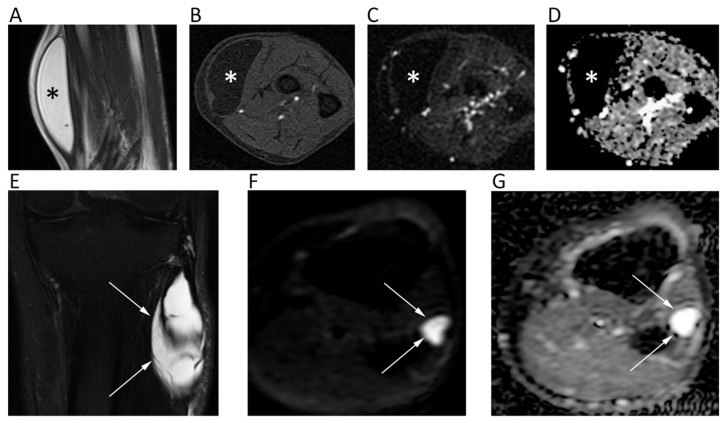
MR images of an intramuscular lipoma of the forearm (A–D) and a perineural ganglion cyst of the leg (E–G) of two different patients. The lipoma (asterisk) shows a homogeneous high signal on coronal T2w (A) and a low signal on axial fat-suppressed T1w (B), *b* = 800 DWI (C); the corresponding ADC map (D). Note the absence of diffusion restriction and the intrinsically low ADC values of this benign lipomatous lesion. The ganglion cyst (arrows) displays high signal intensity on coronal fat-suppressed T2w (E), axial *b* = 800 DWI (F), and axial ADC map (G). High *b*-value DWI shows high signal due to T2 shine-through effect; the ADC map confirms the absence of diffusion restriction and the cystic nature of the lesion (ADC: 2.603 × 10^−3^ mm^2^/s).
